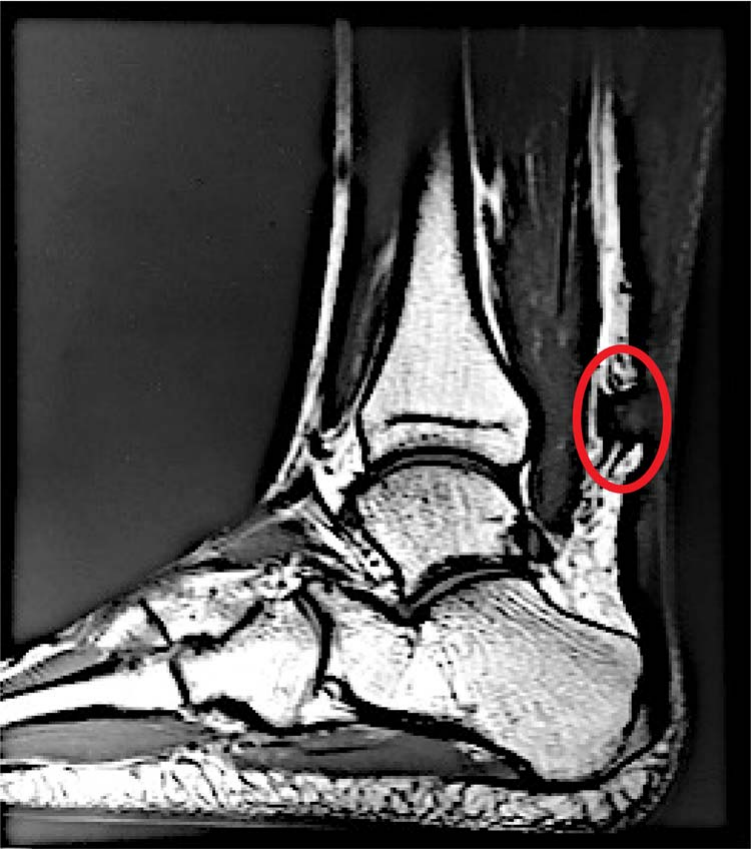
A sagittal view of the MRI of patient’s left Achilles demonstrates an acute, complete, Achilles rupture (circled in red) ~4 cm above the calcaneal insertion.
